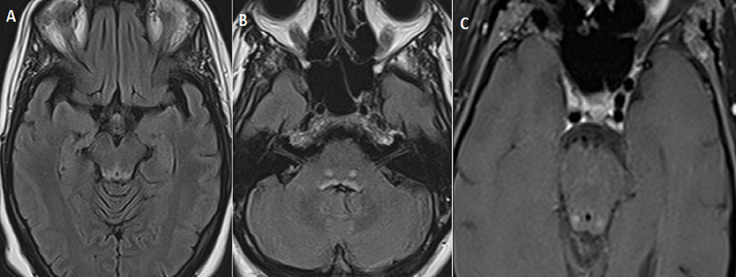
(avant traitement) A) séquence axiale FLAIR: hypersignal bilatéral et symétrique en périaqueducale des corps mamillaires et des colliculi; B) séquence axiale FLAIR: hypersignal bilatéral et symétrique des noyaux dorsaux du pont; C) séquence axiale T1 SE après injection de gadolinium: rehaussement nodulaire des colliculi
